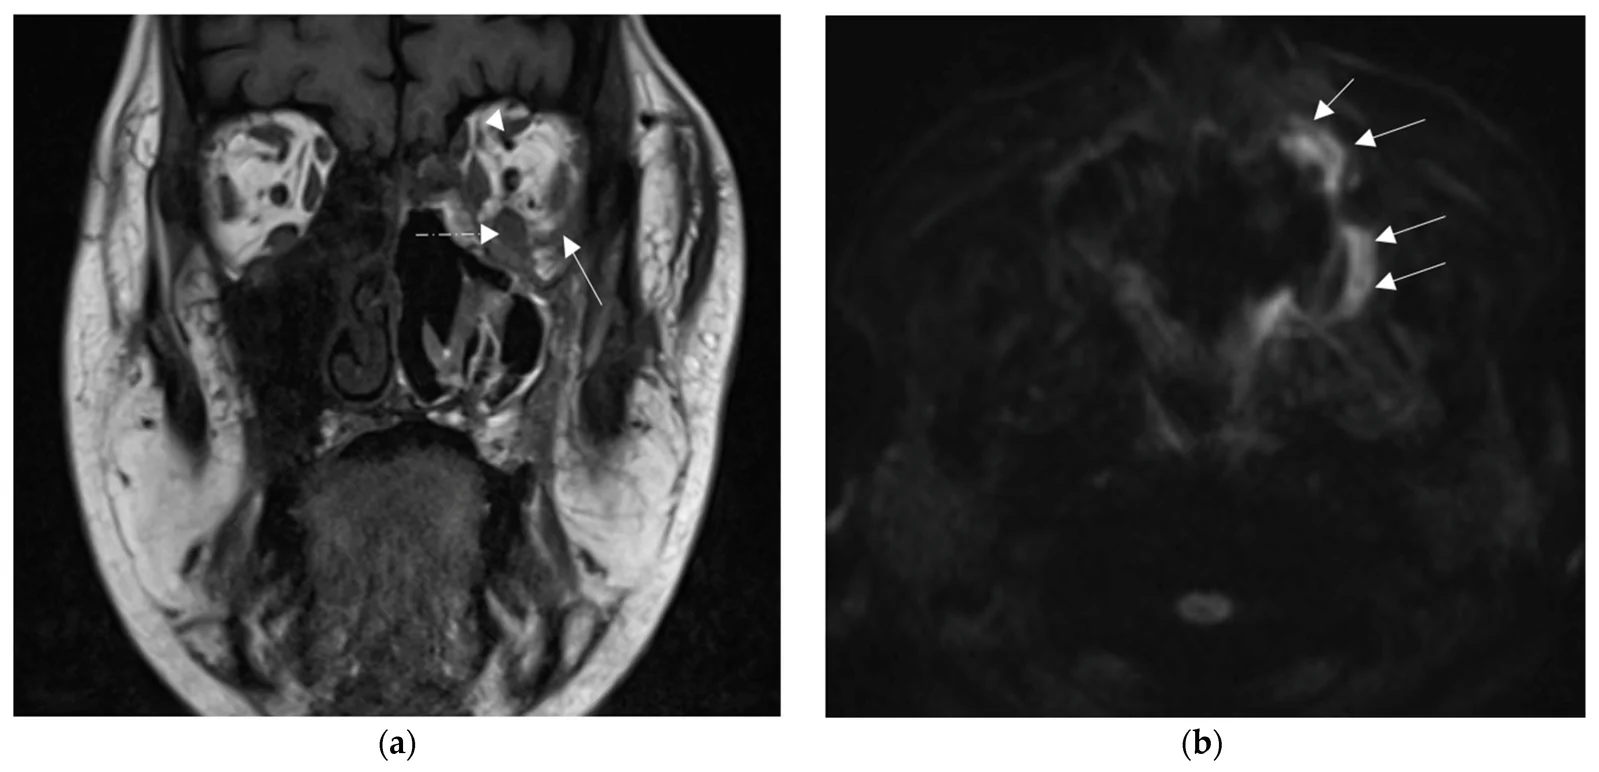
(a) Coronal T1 non-fat-saturated pre-gadolinium injection MRI sequence of the head. There has been interval left-sided endoscopic sinuonasal surgery for evacuation of the sinusitis. Persistent diffuse fat stranding in the left post-septal orbital space (white arrow) with slight enlargement of the extraocular muscles (white dashed arrow). The left superior ophthalmic vein is not dilated (white arrow head). (b) Axial diffusion weight sequence of the brain at the level of the floor of the maxillary sinuses. There is diffusion restriction in the outer gingiva (white arrows) in keeping with abscess (ADC map isnot shon but demonstrated low signal intensity concluding the positive restriction).
